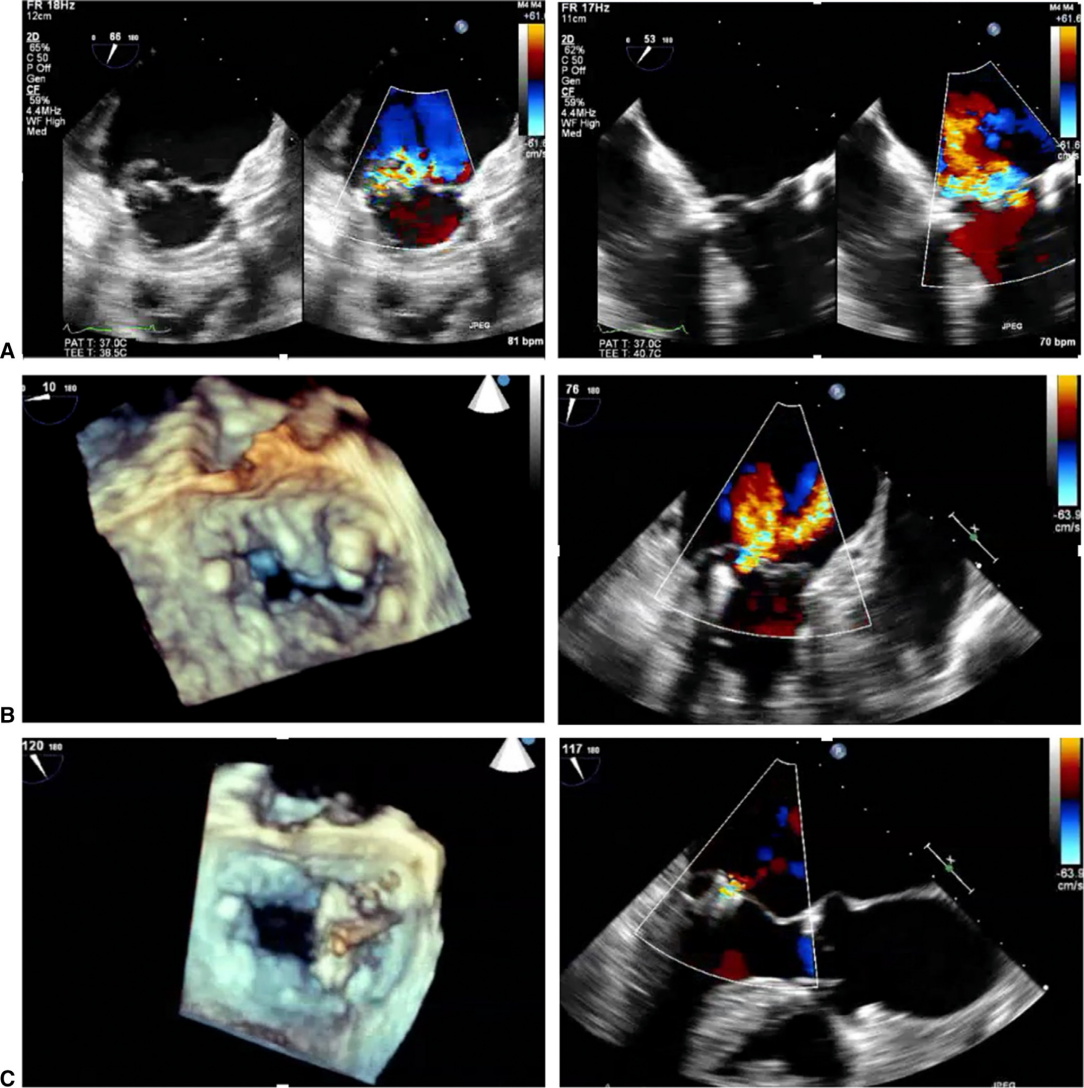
Transcatheter edge-to-edge repair with native MAC. A, Preoperative echocardiogram demonstrating severe MR, flail P3 segment, and moderate focal posterior MAC. B, 3D (*right panel*) and 2D echocardiogram (*left panel*) demonstrating placement of first MitraClip. C, 3D (*right panel*) and 2D echocardiogram (*left panel*) demonstrating placement of second MitraClip.
